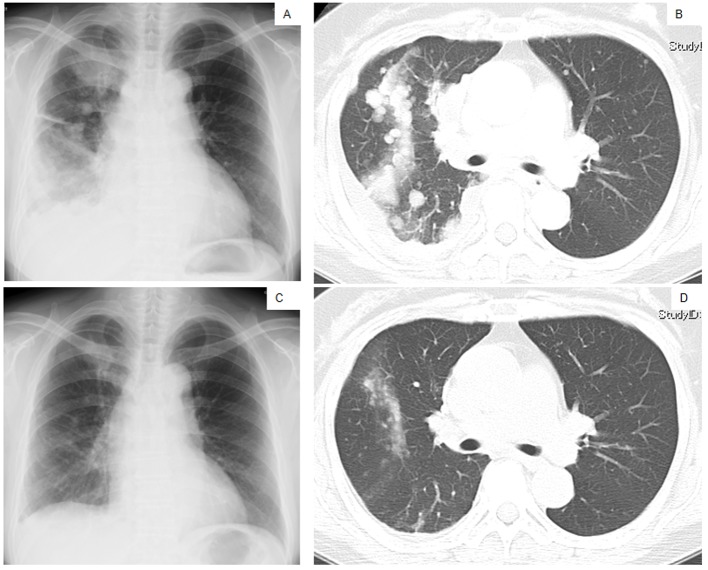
Chest X-ray films (A, C) and a CT scan of the middle lung fields (B, D) taken in April 2010 before first-line chemotherapy using carboplatin plus weekly paclitaxel (A, B), and taken in May 2010 after the second course of the first-line chemotherapy (C, D).
